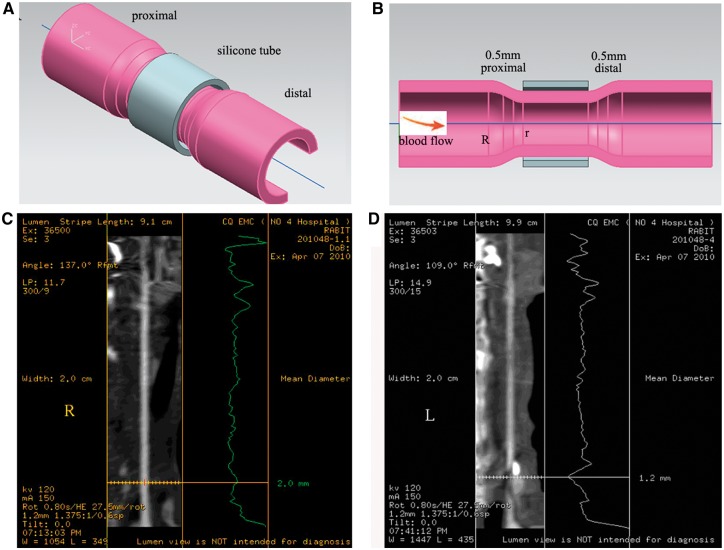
The model of perivascular collar placement. A stenosis of the vessel presents the stenosis of the plaque intrudes into the lumen (A and B). computed tomography (CT) scanner detects the diameter of the vascular lumen in the control right (C) and left (D) carotid artery
